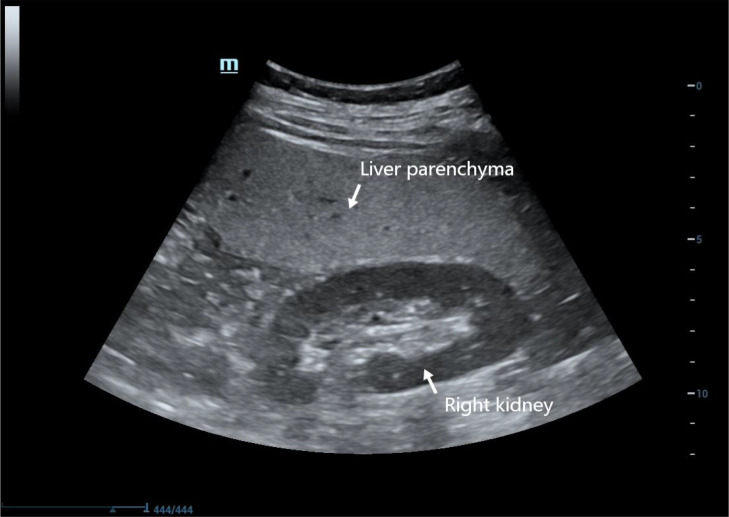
Conventional B-mode ultrasound image of the liver. The grayscale image demonstrates typical hepatic parenchyma (annotated) adjacent to the right kidney (annotated), allowing visual comparison of echogenicity between the liver and renal cortex, a commonly used reference in ultrasound assessment of hepatic steatosis. While conventional B-mode imaging provides important anatomical context, it does not offer direct quantitative assessment of hepatic fat, fibrosis, or microstructural tissue properties.
